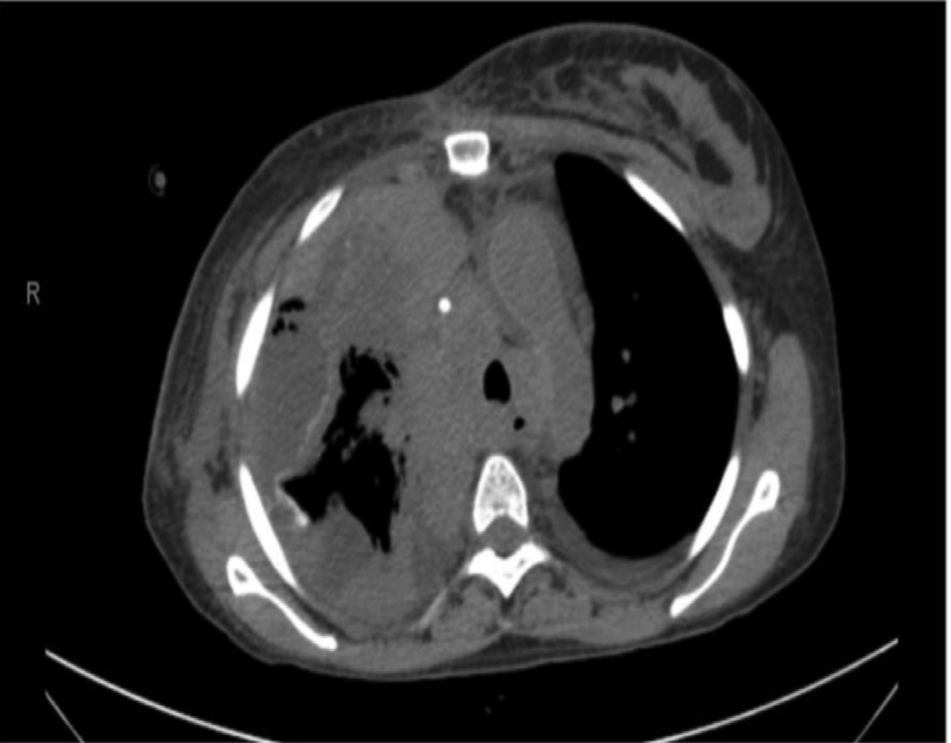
Empyema post-pleurodesis on chest CT scan. Caption: Chest CT scan (axial) after talc pleurodesis, showing a right malignant pleural effusion loculated, pleural calcification secondary to talc, pleural thickening, and intervening gas suggestive of empyema.
